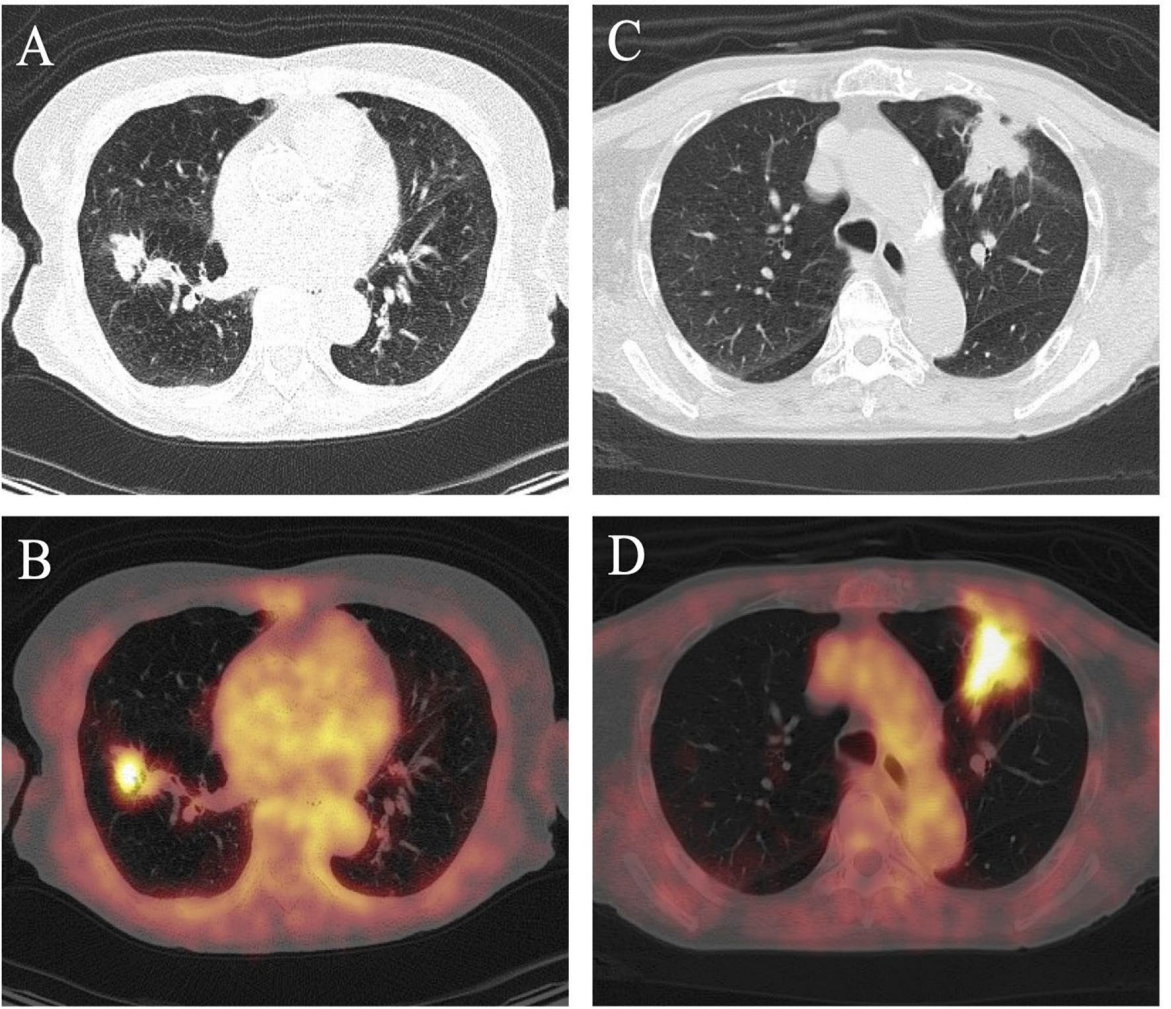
Representative CT and fused PET/CT images of NSCLC and RP. (A) CT image shows mass in right middle lobe of 79-year-old male with NSCLC. (B) Axial fused PET/CT image shows high ^18^F-FDG uptake (SUV_max_, 4.32) and heterogeneous ^18^F-FDG distribution (GLRLM run percentage, 0.81; NGTDM coarseness, 0.0068). (C) CT Image of 77-year-old male with RP shows mass-like attenuation in left upper lobe. (D) Axial fused PET/CT image shows high ^18^F-FDG uptake (SUV_max_, 3.75) and homogeneous ^18^F-FDG distribution (GLRLM run percentage, 0.65; GLSZM coarseness, 0.0017).
